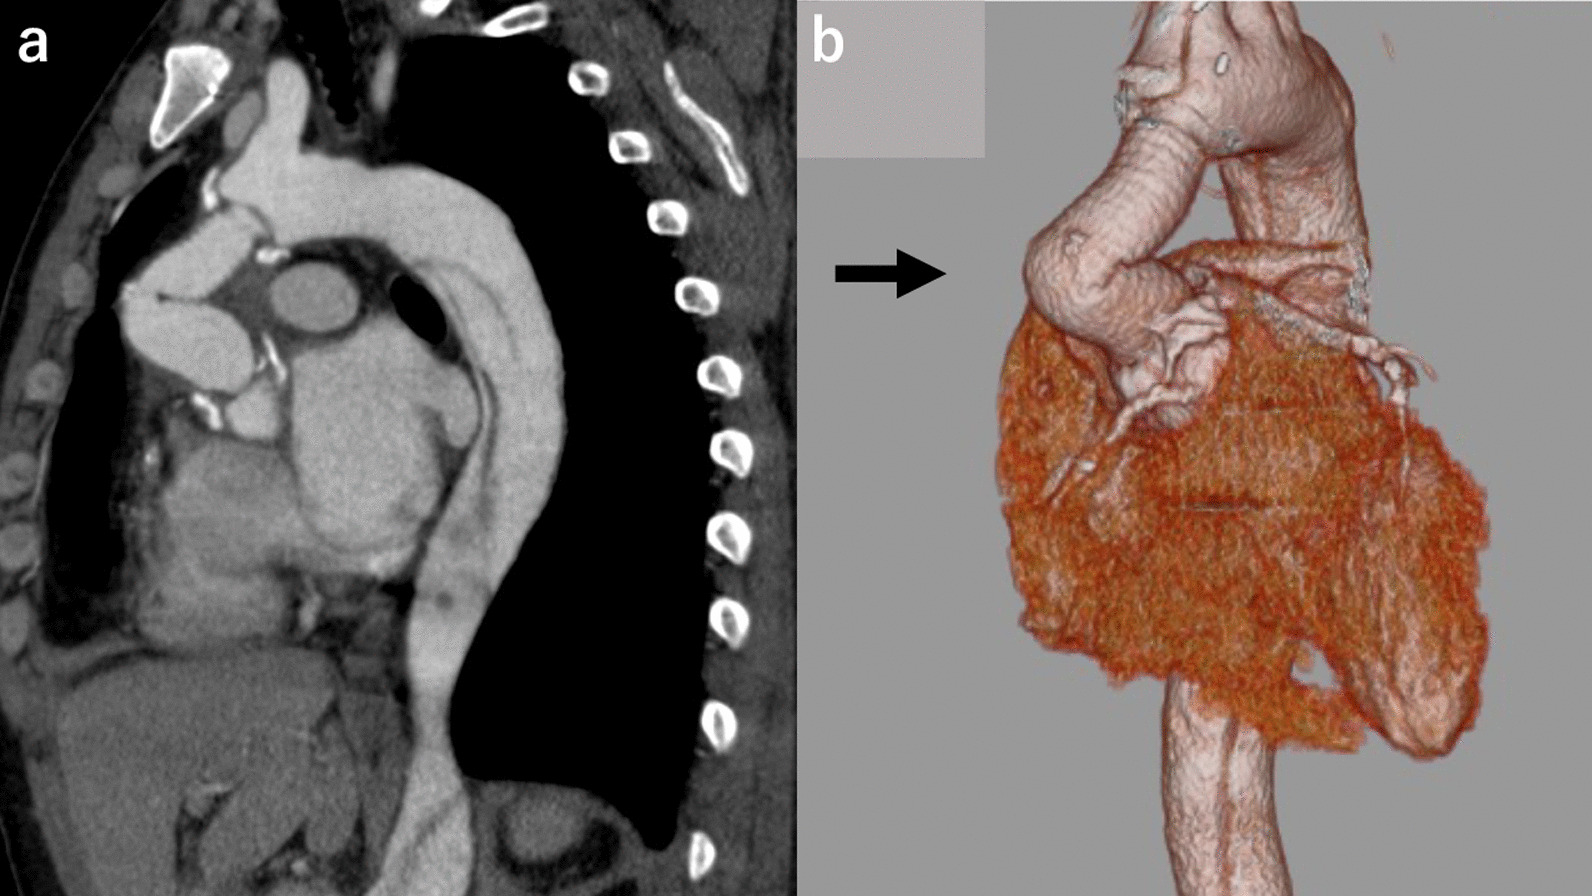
Chest CT scan before redo surgery showed severe kinking of the graft (arrow) approximately 35 mm from the proximal anastomosis. a saggital view. b 3D-CT
